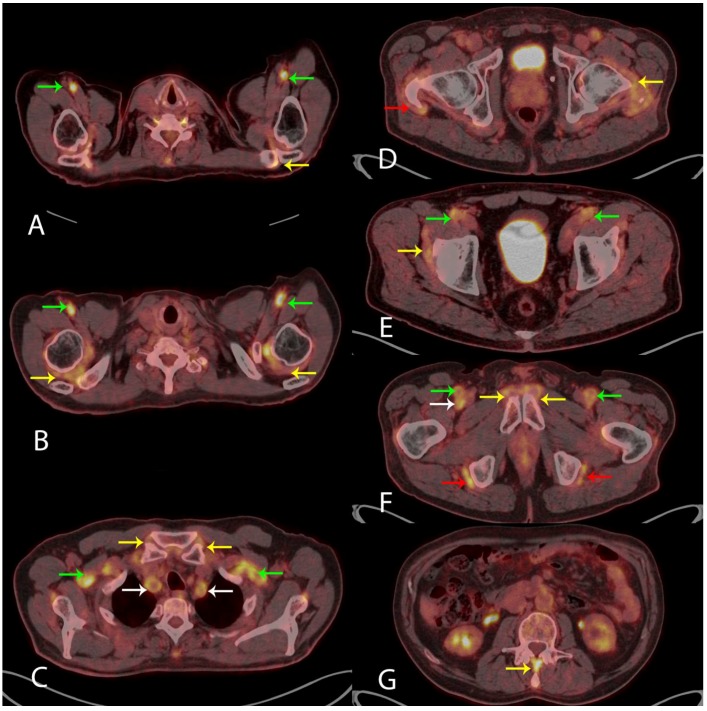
Visually measuring the ^18^F-FDG uptake in nine anatomical sites when evaluating the PET/CT scan for the diagnosis of PMR has been suggested [5,6]. These sites include: acromioclavicular joints (A) axial fused PET/CT (yellow arrow, left side shown), the high ^18^F-FDG uptake in the axillary arteries indicates LVV (green arrows); shoulder joints (B) axial fused PET/CT (yellow arrows), LVV in axillary arteries (green arrows); sternoclavicular joints (C) axial fused PET/CT (yellow arrows), LVV in subclavian arteries (green arrows) and common carotid arteries (white arrows); hip joints (D) axial fused PET/CT (yellow arrow, left side shown) and greater trochanter (D) axial fused PET/CT (red arrow, right side shown); the two iliopectinal bursae (E) axial fused PET/CT (yellow arrow, right side shown), LVV in common femoral arteries (green arrows); two symphysis pubis enthesis (F) axial fused PET/CT (yellow arrows) and two ischial tuberosities (F) axial fused PET/CT (red arrows), LVV in superficial femoral arteries (green arrows) and right profound femoral artery (white arrow); and interspinous ligaments (G) axial fused PET/CT (yellow arrow), respectively. The specificity of ^18^F-FDG PET/CT in the diagnosis of PMR is 95% given that the ^18^F-FDG uptake is above the uptake in the liver in ≥6 anatomical sites [5]. In our case, the ^18^F-FDG uptake met this criterion in all nine anatomical sites (not quantitatively shown). No malignancy was visualized. Based on the clinical information and the results of the ^18^F-FDG PET/CT, the patient was treated with 60 mg of Prednisolone resulting in the normalization of blood tests and rapid remission of symptoms. Our case shows that ^18^F-FDG PET/CT is a strong supportive imaging tool in the diagnosis of PMR and LVV. ^18^F-FDG PET/CT can be used where malignancy is a differential diagnosis, as proposed in the newest European League Against Rheumatism (EULAR) recommendations [7].
